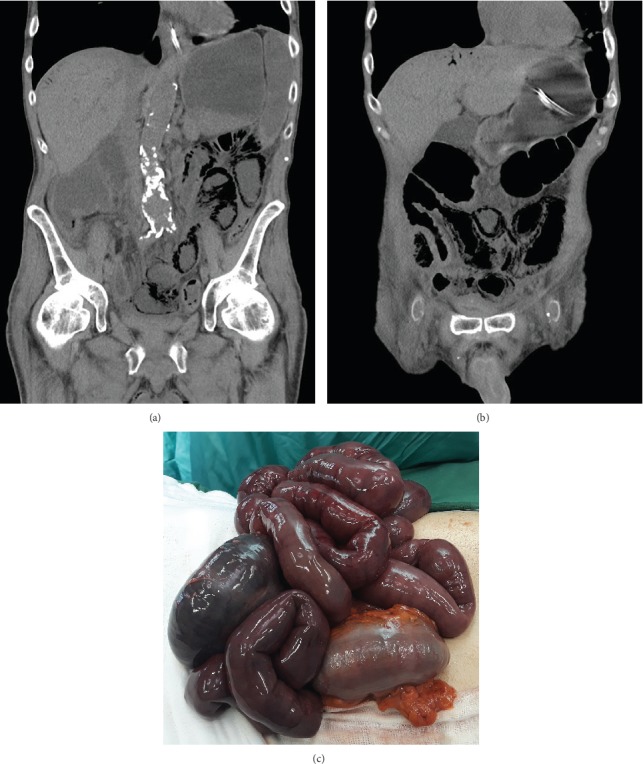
(a, b) Multiphase computed tomographic angiography (angio-CT) of the abdominal cavity and pelvis in a patient with acute mesenteric ischemia without symptoms of peritonitis. Signs of intestinal obstruction and pneumatosis of the small and large intestinal walls due to an embolism of the superior and inferior mesenteric arteries. (c) Intraoperative view of the same patient. Widespread necrosis of the walls of the small intestine and colon was revealed during exploratory laparotomy. Resection was not performed due to the advancement and extent of necrotic changes.
